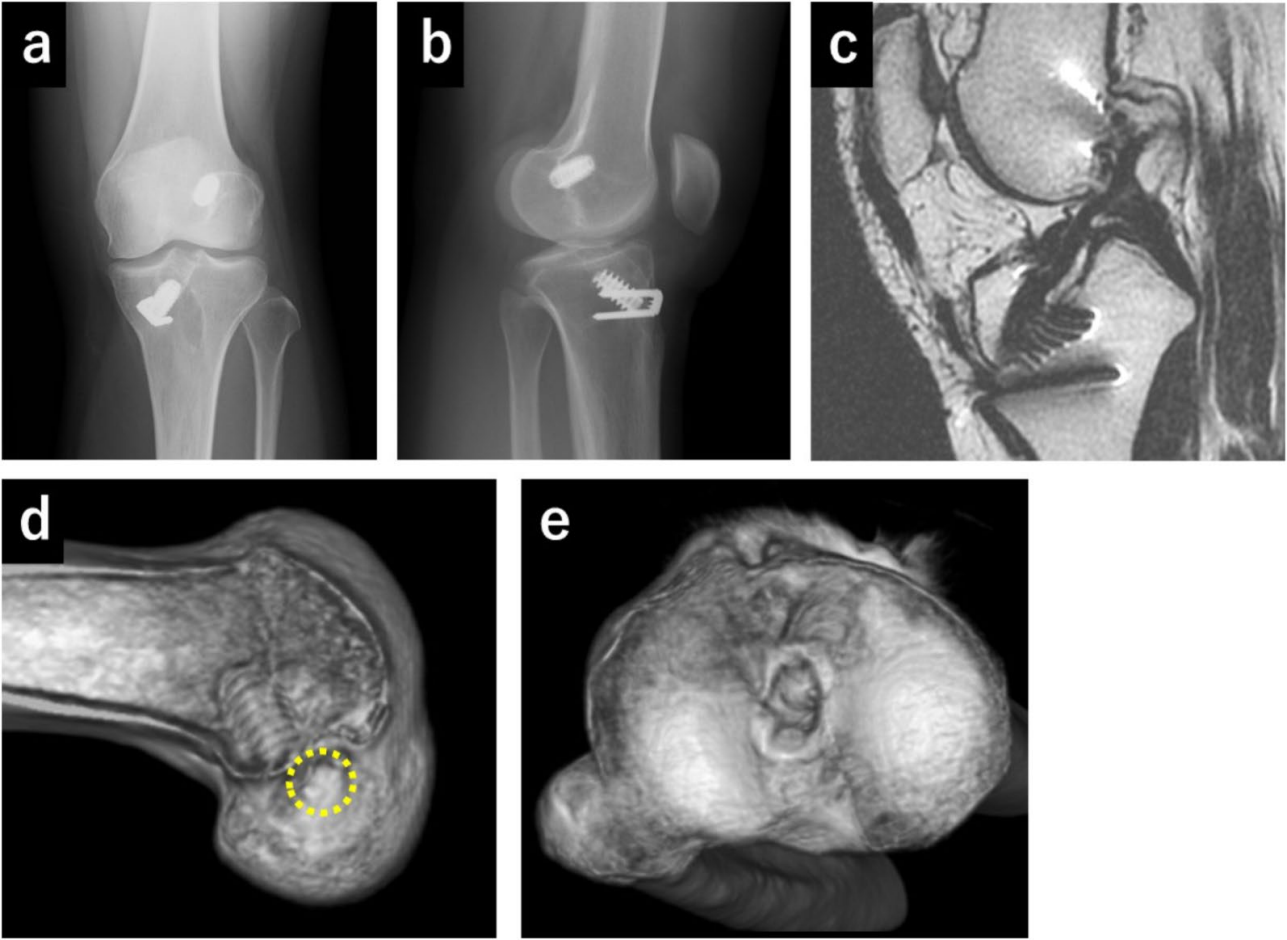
X-ray, magnetic resonance imaging (MRI), and computed tomography (CT) scans in case 2 (a–e). (a) Anteroposterior view of the X-ray. (b) Lateral view of the X-ray. (c) T1-weighted MRI image of the sagittal plane, showing a partially residual reconstructed anterior cruciate ligament. (d) Three-dimensional (3D) CT image of the femoral tunnel position of the left knee. The yellow dot circle shows the aperture of the femoral tunnel. CT shows nonanatomical tunnel position (anterior and distal). (e) 3D CT image of the tibial tunnel position, showing a relatively posterior tunnel position
